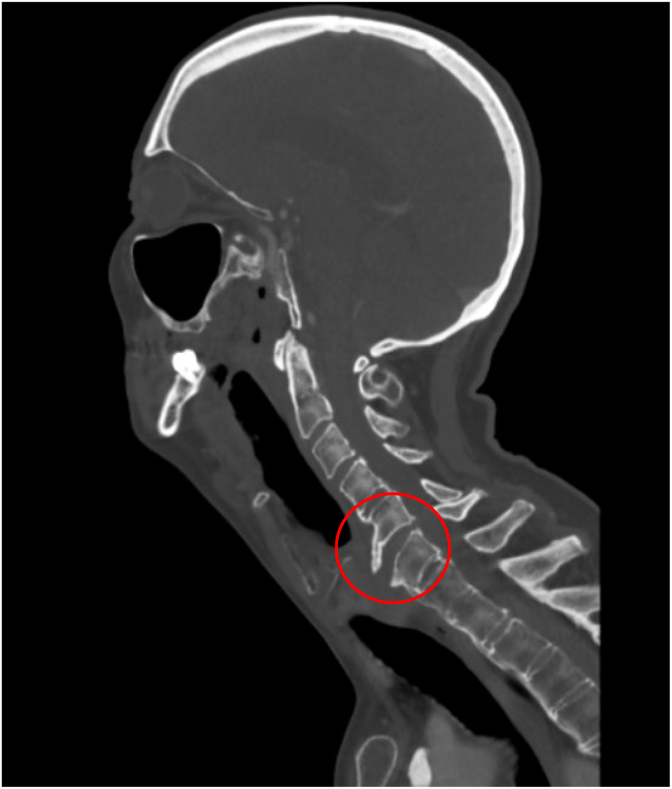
Sagittal stroke CT angiogram head and neck demonstrating separation of a C5-6 osteophyte (red circle) previously stabilizing C-spine.
